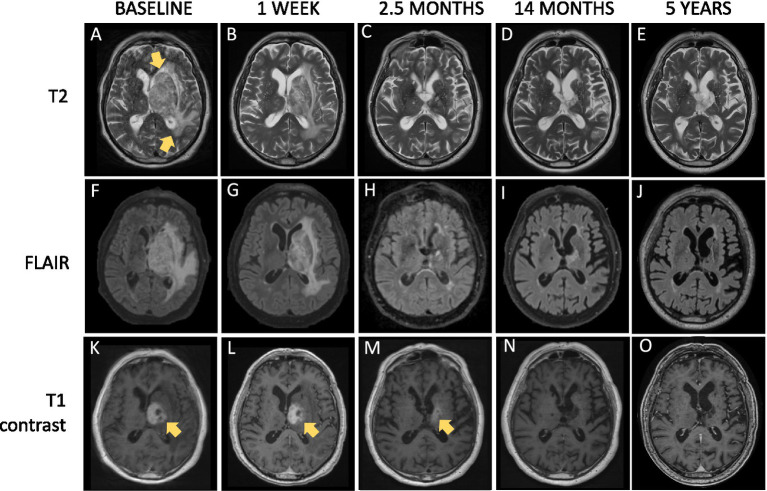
Magnetic resonance imaging (MRI) sequences including T2-weighted (A–E), fluid-attenuated inversion recovery (FLAIR) (F–J), and contrast-enhanced T1-weighted (K–O) imaging showcasing the acute phase and follow-up over 5 years. At baseline, a contrast-enhancing tumor measuring 3.3 × 3 × 3.5 cm (K) with central necrosis and blood products was observed in the basal ganglia, resulting in compression of the left lateral ventricle. There were marked peritumoral hyperintense changes on T2 (A) and FLAIR (F) extending to the midbrain, pons, through the left cerebellar peduncle to the left cerebellar hemisphere, as well as the entire left frontal lobe, parietal lobe, and part of the left temporal lobe. In (A), yellow arrows indicate the extent of the lesion and edema on T2 at baseline. Yellow arrows are also used to highlight the central contrast enhancement within the lesion in (K,L,M).
